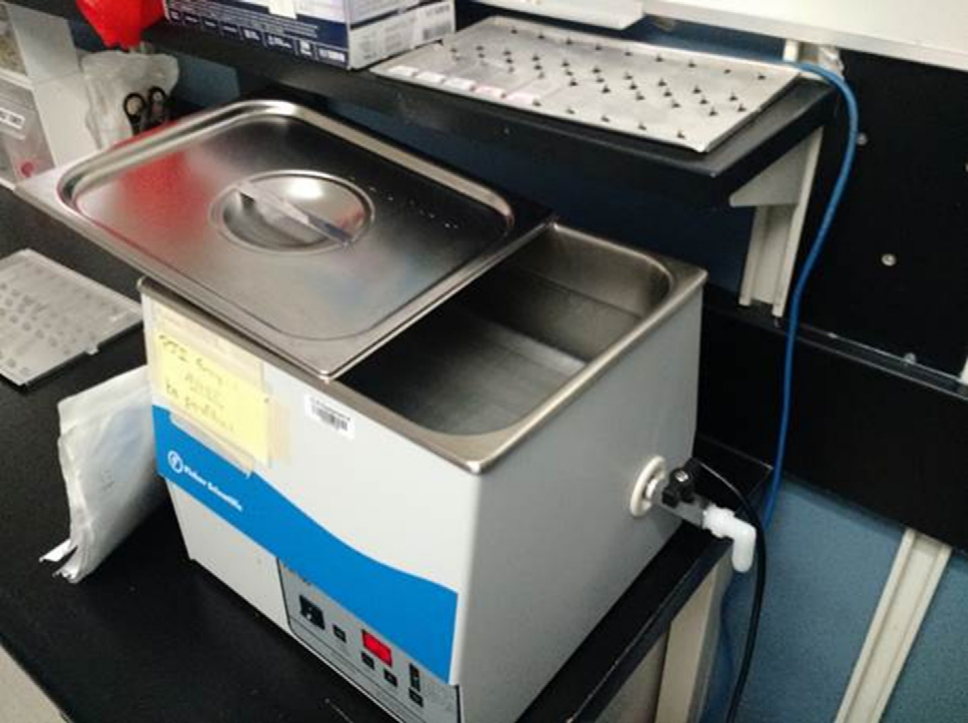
Ultrasonicator device used to disrupt bacterial biofilm on prosthetic and implant devices. The prosthetic device is placed in a container filled with saline and then placed in this waterbath where ultrasound waves are directed at it.
